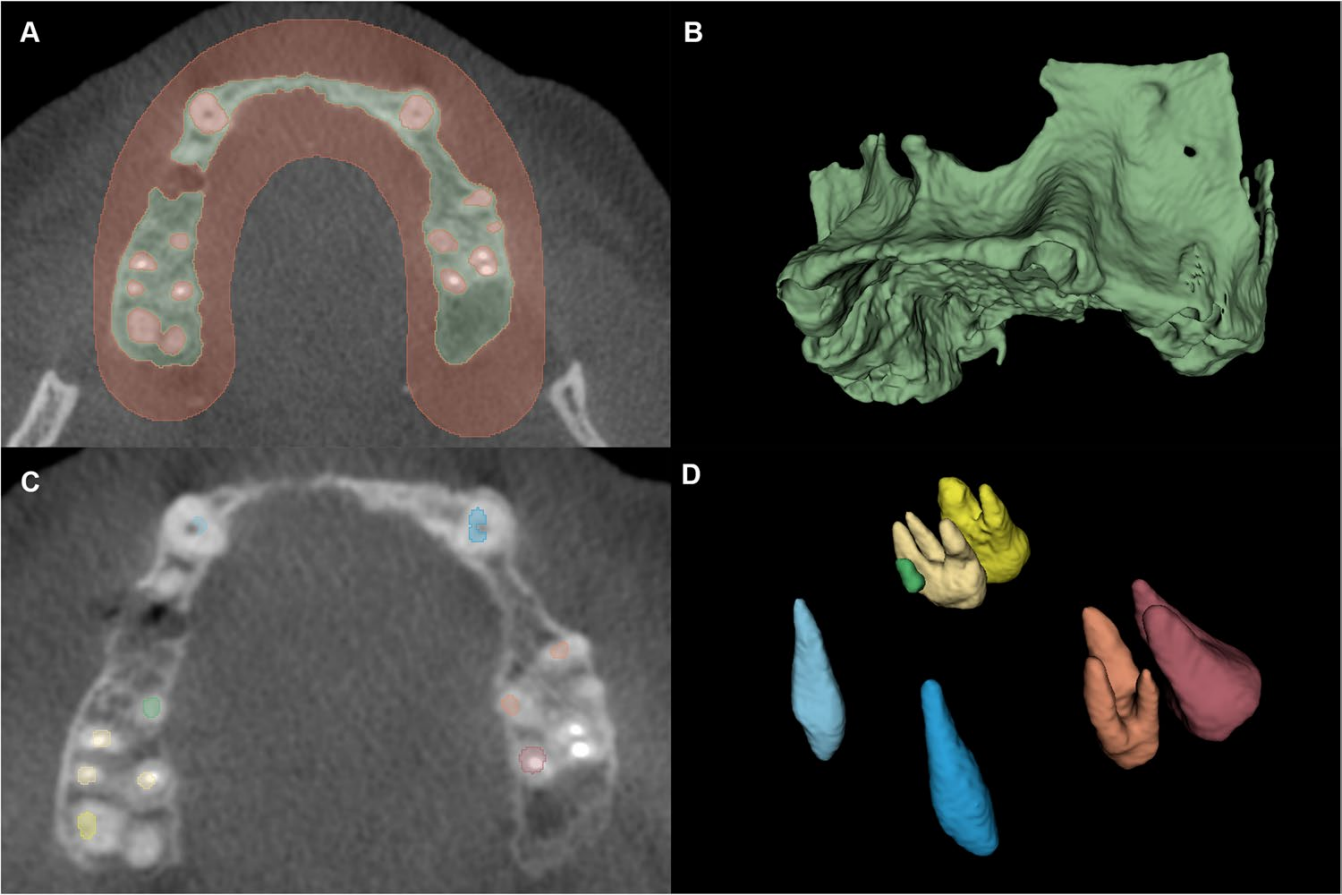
Presentation of the semi-automatic segmentation method. A Alveolar bone and background input labels generated on the axial view of the CBCT scan 3D model acquired via semi-automatic segmentation (green, alveolar bone; red, background). B 3D model of the alveolar bone following region-growing segmentation. C Labels generated for each tooth separately serves as the input of the watershed segmentation. D 3D surface representation of label maps following the application of the watershed segmentation method
